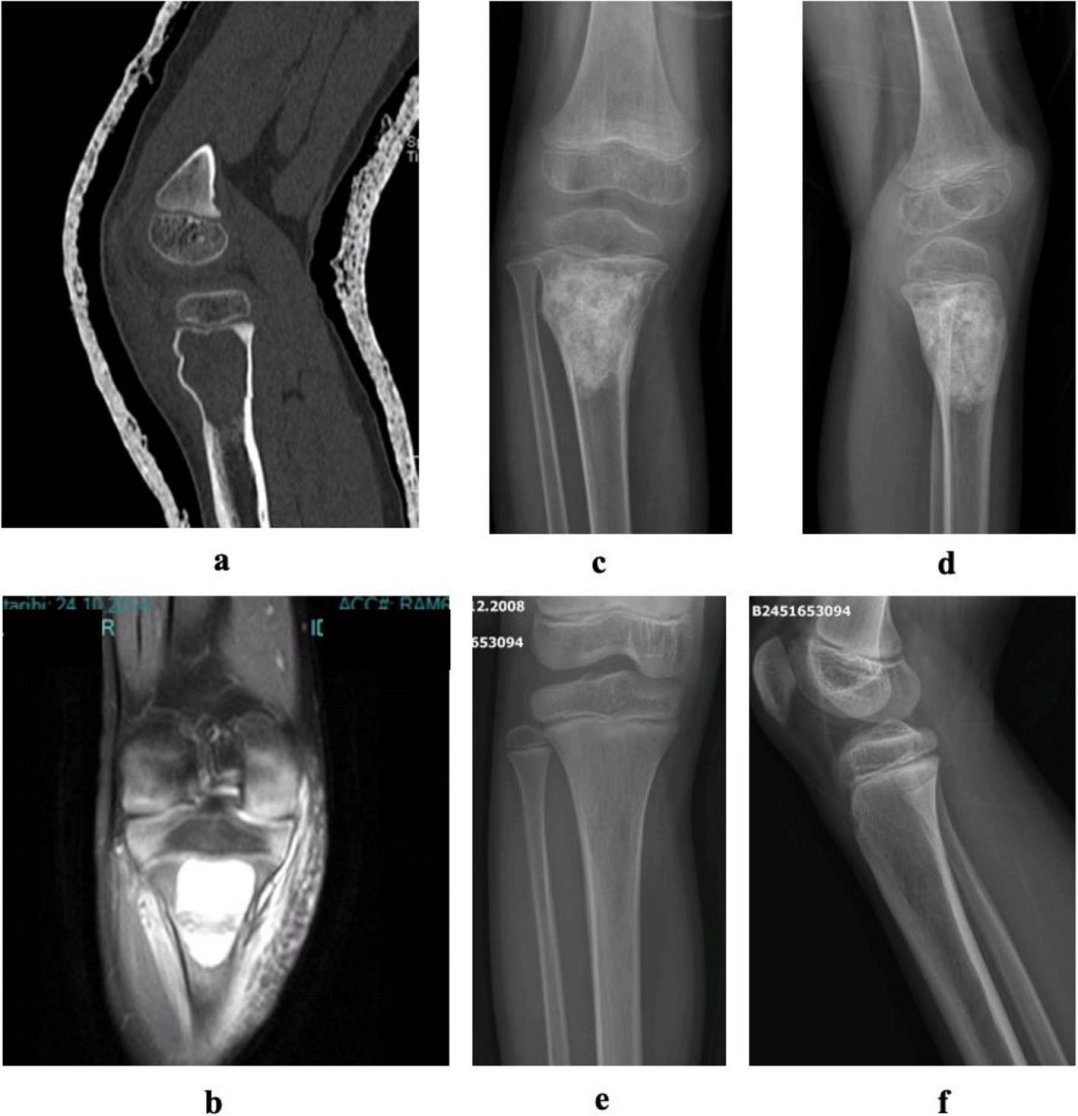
a CT section of the cyst. b MRI section of proximal tibial cyst. c Early postoperative AP radiograph. d Early postoperative lateral radiograph. e Postoperative 8th year AP Radiograph. f Postoperative 8th year lateral radiograph
